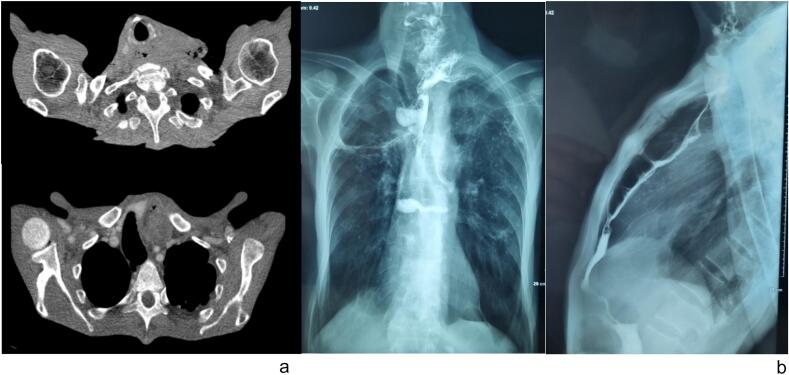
(a) CT view showing distension of the oesophageal plasty at the left cervical level with bubbles of extra-digestive areas without extravasation of contrast. (b) Barium swallow showing heterogeneous aspect of the colonic esophageal plasty with 2 blind fistulas of the left cervico-thoracic junction
